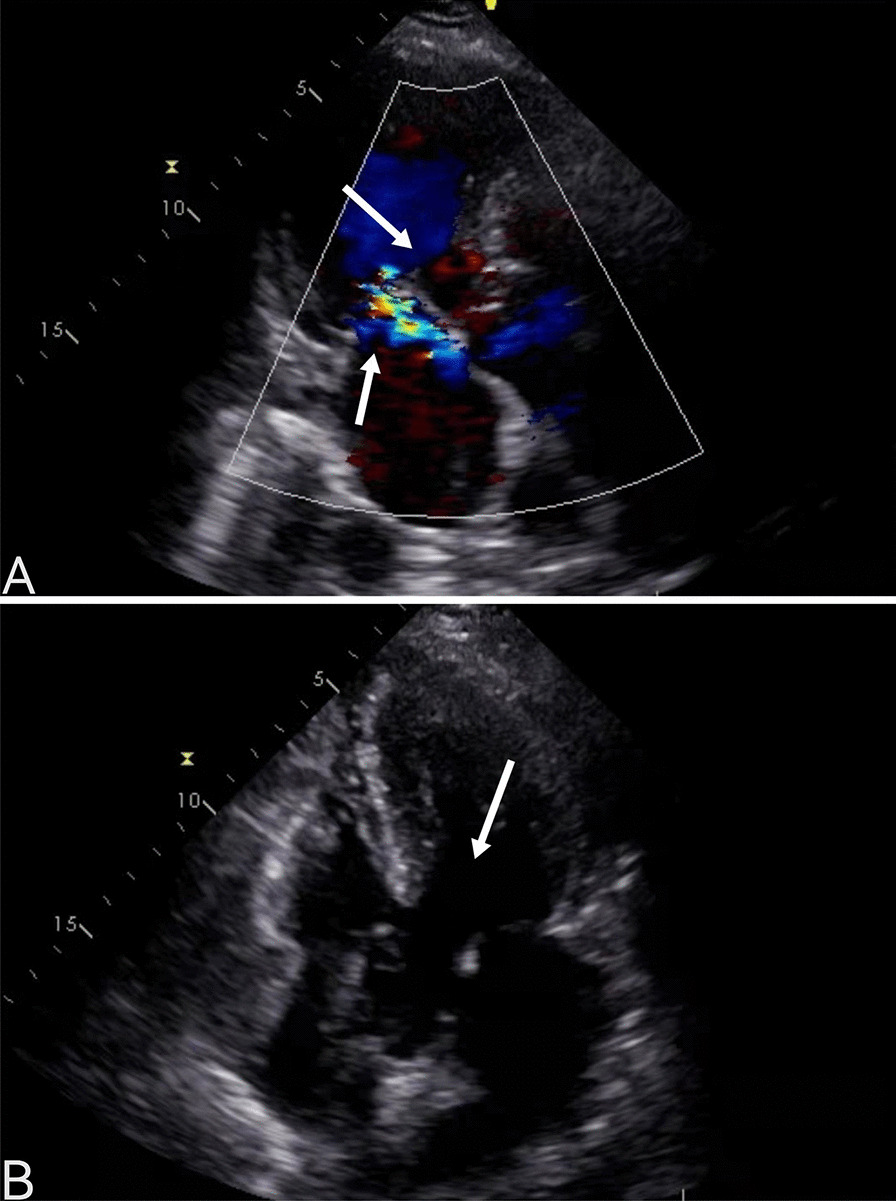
Echocardiography postoperatively.The mitral valve regurgitation was mild (A, the arrow below). LVOT blood flow is unobstructed (A, The arrow above). The SAM sign disappeared (B, arrow)
